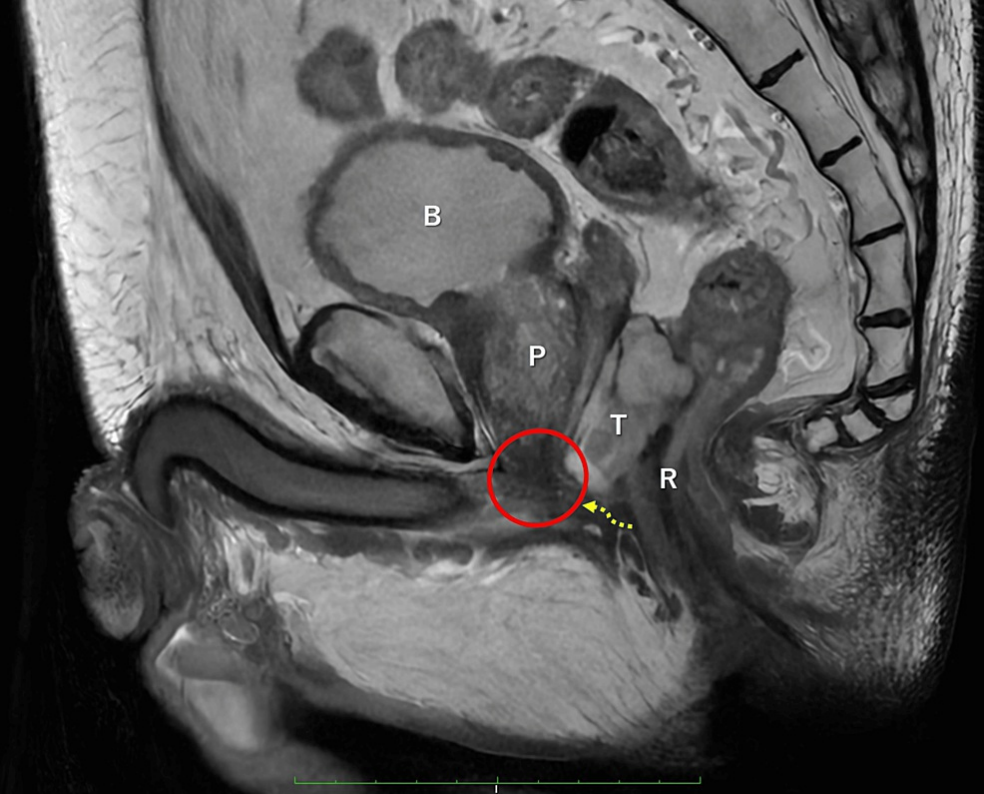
Strategy for surgery, avoiding urethral injury On sagittal MRI, the caudal end of the tumor was located at the level of the urethra, where the dissection line was obscured by the complex anatomy, particularly, the rectourethral muscle (red circle). Therefore, immediate dissection of this area would have increased the risk of straying into the urethra or tumor. B, bladder; P, prostate; T, tumor; R, rectum
